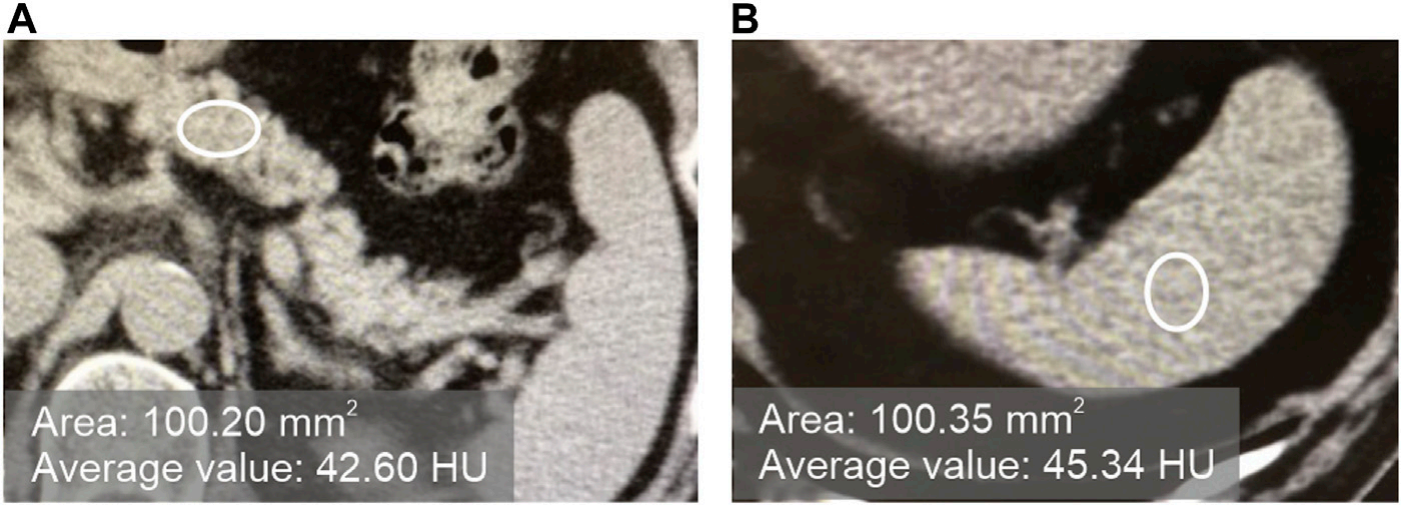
Measurement of the CT values of the pancreas and spleen in plain CT. [(A): pancreas, (B) spleen].
